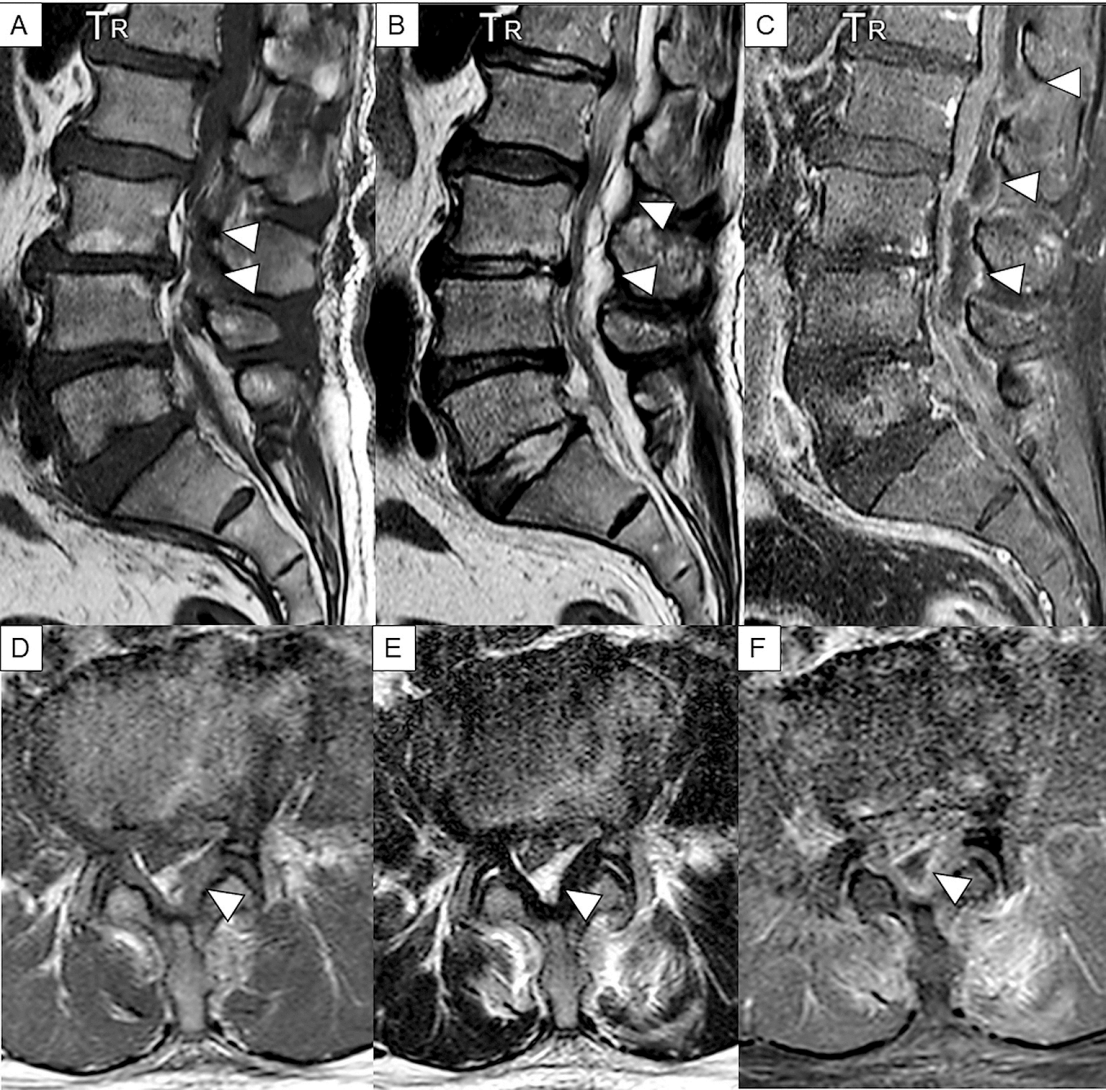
Sagittal and axial views of T1-weighted, T2-weighted, and Gd-enhanced T1-weighted MRI. Extensive epidural phlegmon is noted throughout the lumbar spine in the sagittal view; T1-weighted images show low signal intensity (A), T2-weighted images show high signal intensity (B), and Gd-enhanced MRI shows that a linear structure appears to be a thickened dural surface on the ventral side of the lamina. Acknowledge the occupying lesions inside and capsule-like rims whose edges are strongly enhanced. (C). The axial view at the level of L4 confirms that the dural tube is compressed by posterior SEA in each image (D–E). The enhancement is also observed in the L5 vertebral body and the paraspinal muscles, representing spondylitis and spread of inflammation into the surrounding muscles (C, F). Arrowheads indicate SEA.
